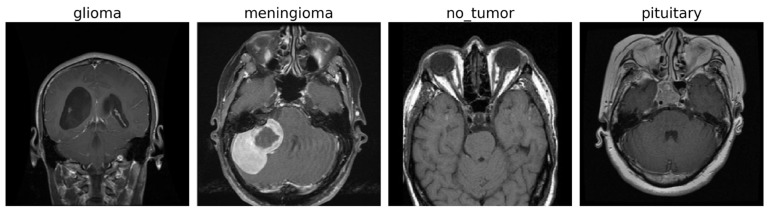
MRI images of brain tumor and normal brain.
